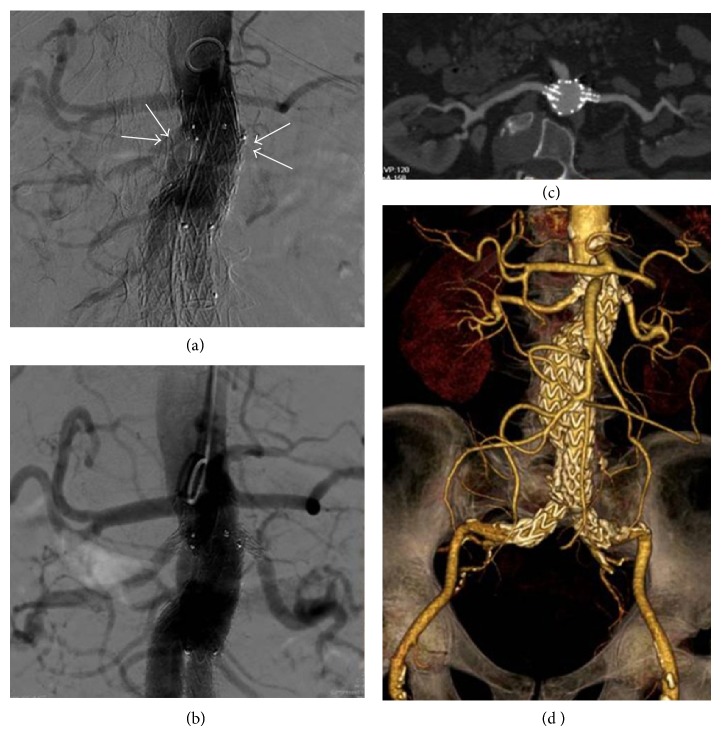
Selective angiography at the proximal extremity of the EG (a) showed the overstenting (arrows) of the origin of both the renal arteries. Complete revascularization after bilateral stenting (b). Follow-up CT-A: complete reperfusion of the parenchyma (c) as well as persistent exclusion of the aneurysm and absence of endoleak (d).
